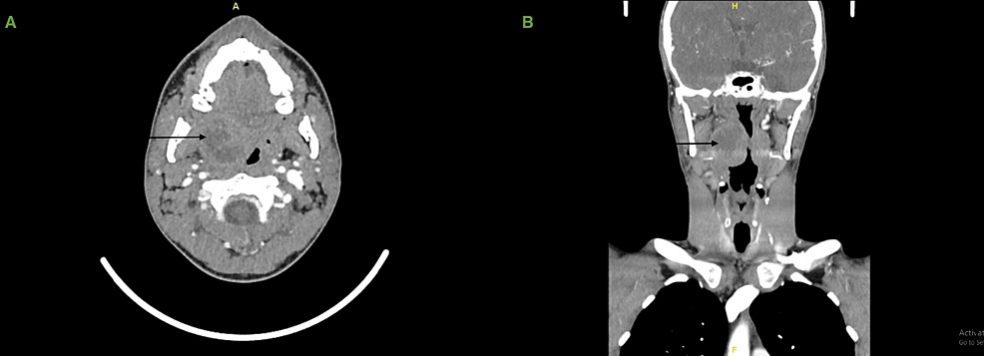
CT of the neck with IV contrast showing a well-defined collection in the right tonsillar region Axial view (A), coronal view (B)
